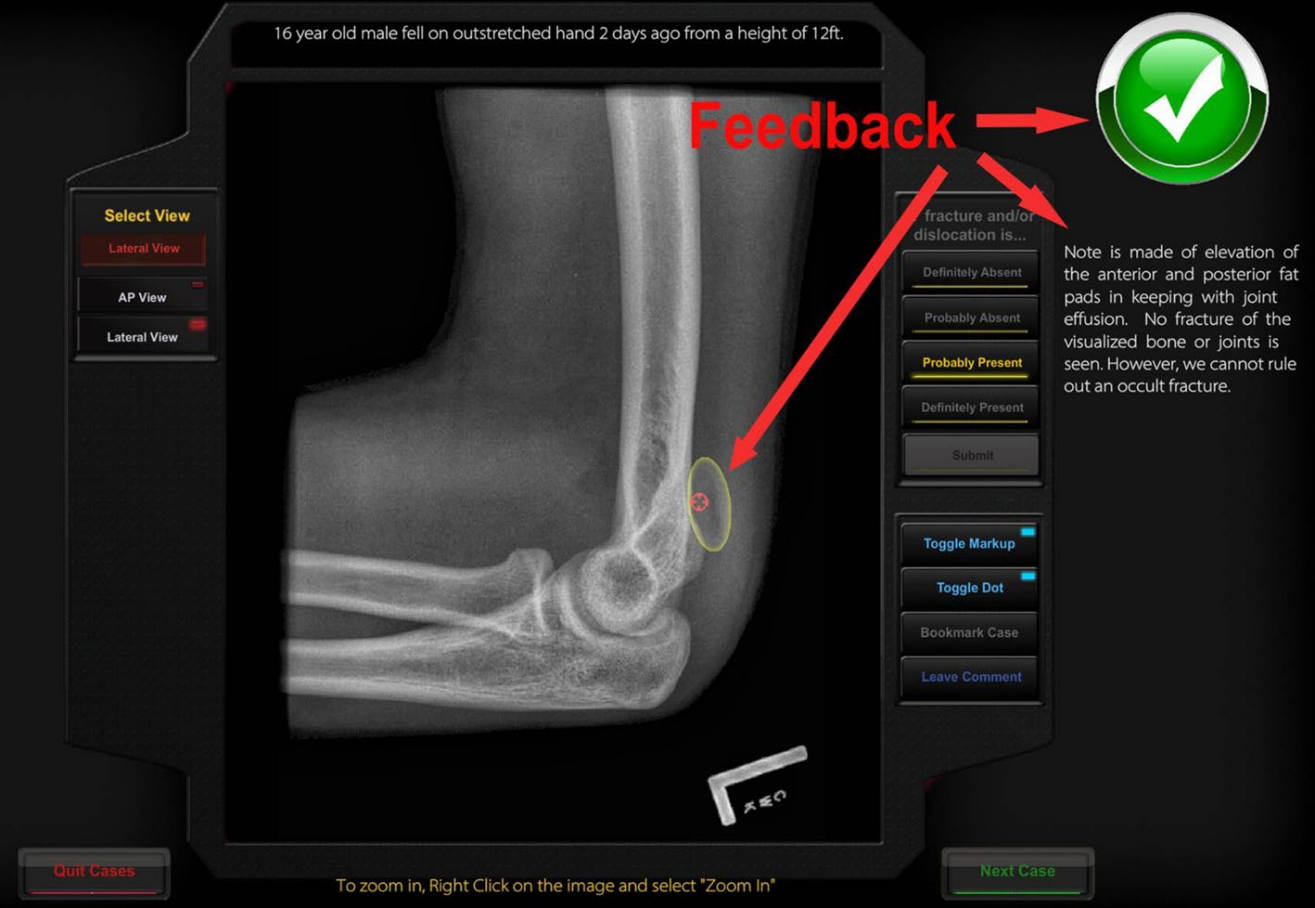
A screen capture from the ImageSim learning system used in the study. Shown is the feedback page demonstrating: a the learner’s assignation of where the fracture lies (red marker) which would have been placed by the learner on a prior screen, on an otherwise unmarked radiograph; b three forms of feedback including overall correctness (green checkmark), text of radiologist report, and yellow target area pre-assigned by an expert radiologist. (Color figure online)
